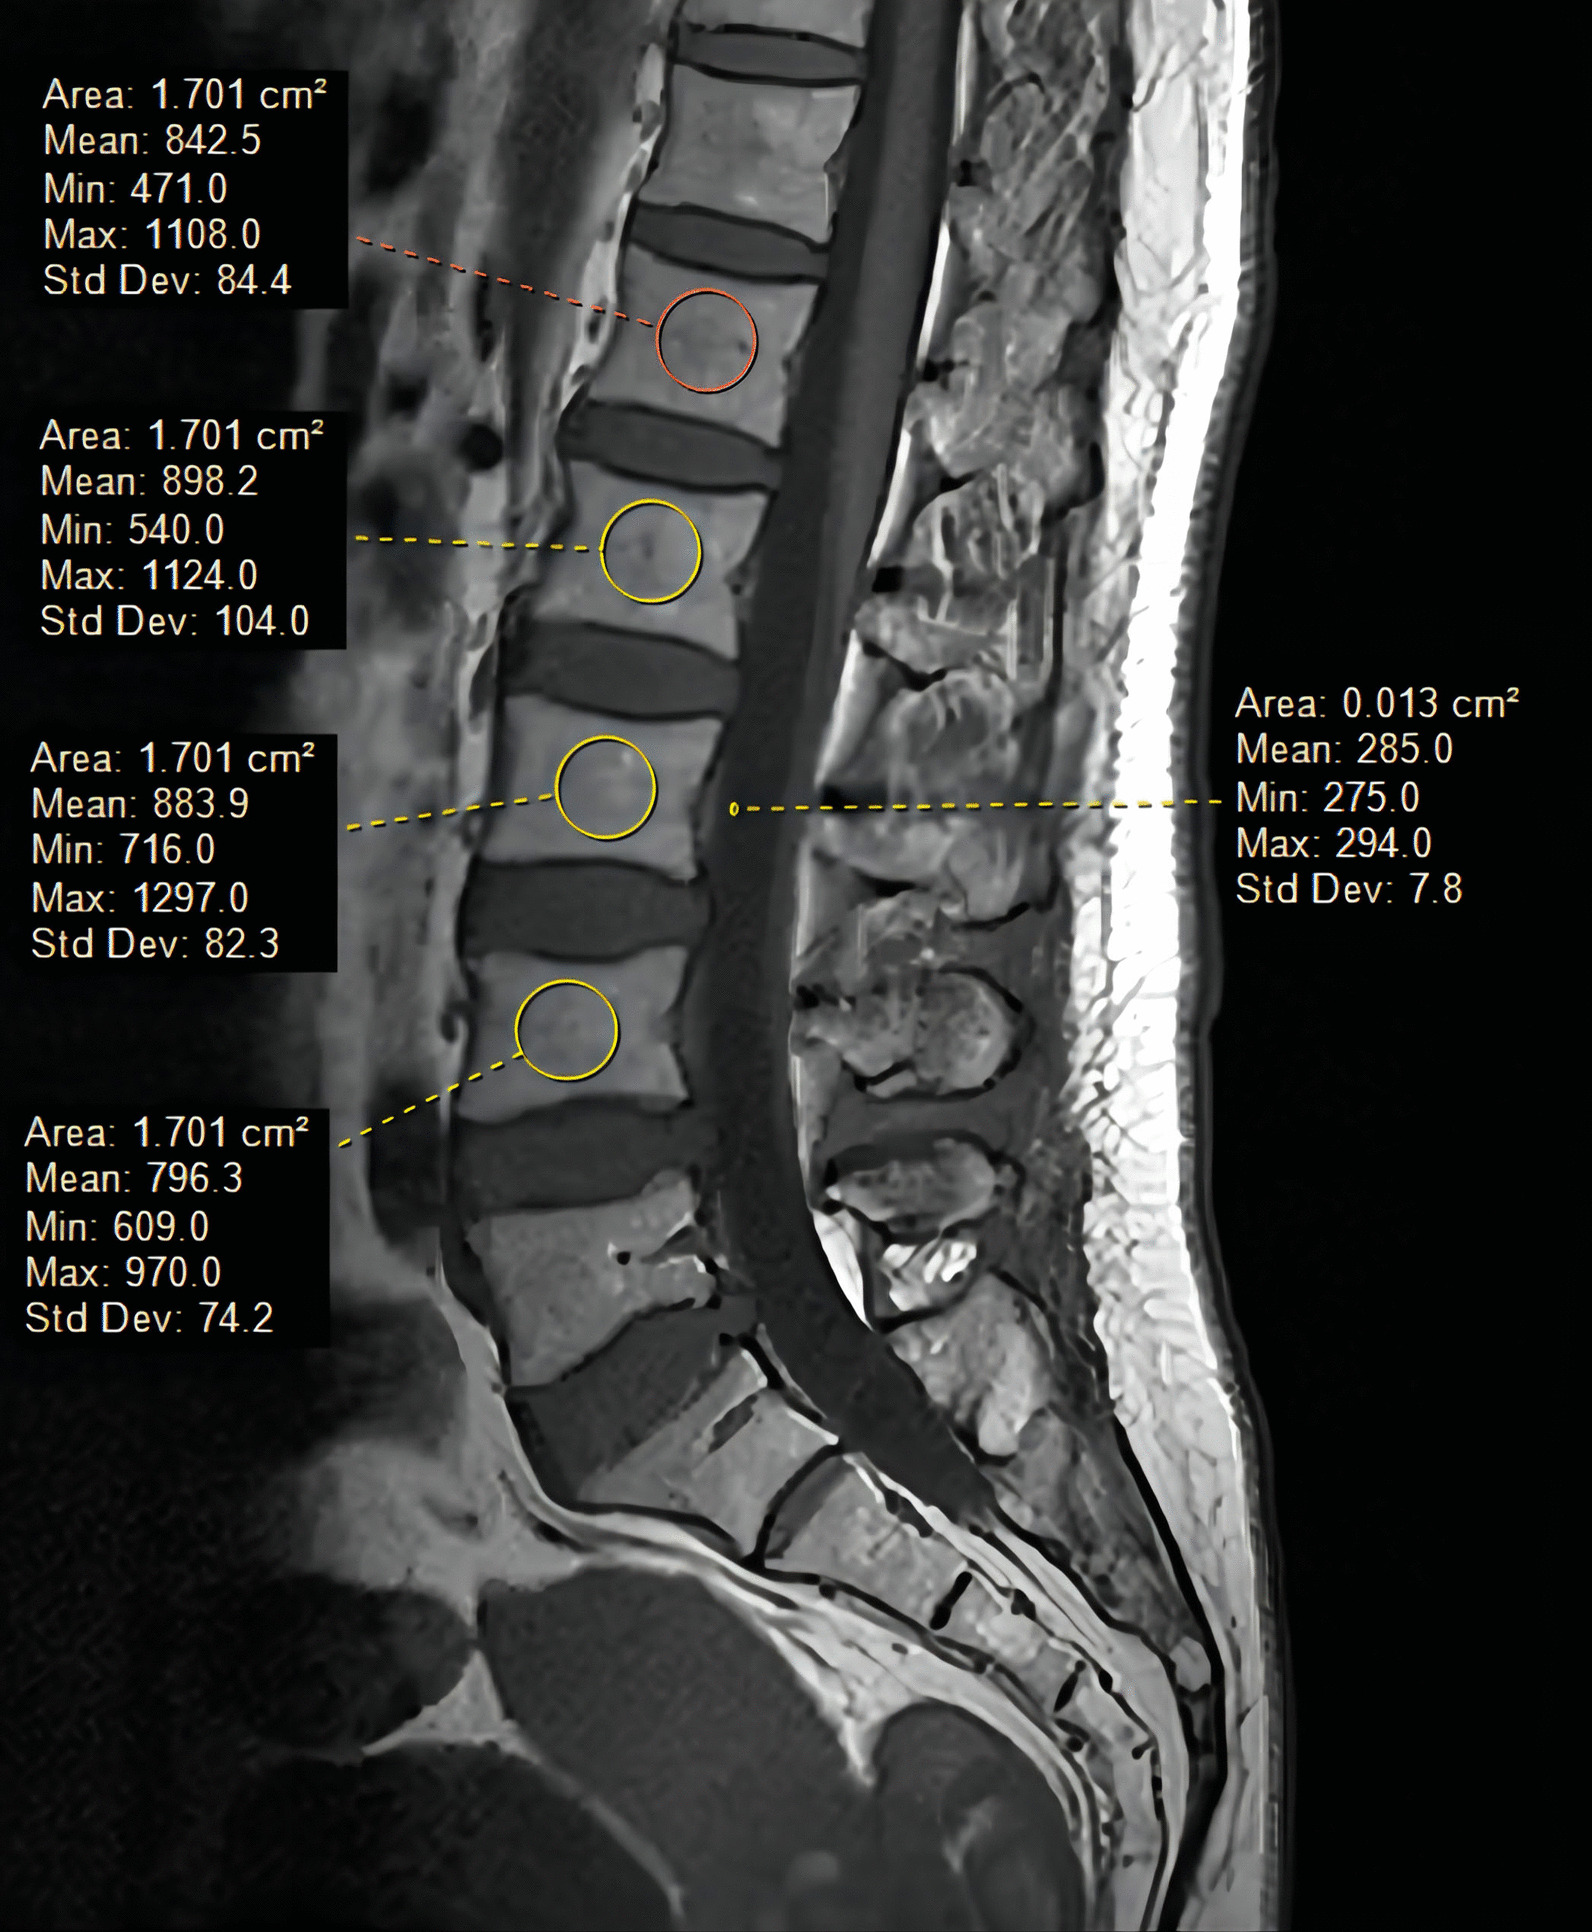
On sagittal non-T1-weighted images, a ring-shaped region of interest was placed in the corresponding region to display the SI to obtain the VBQ score
